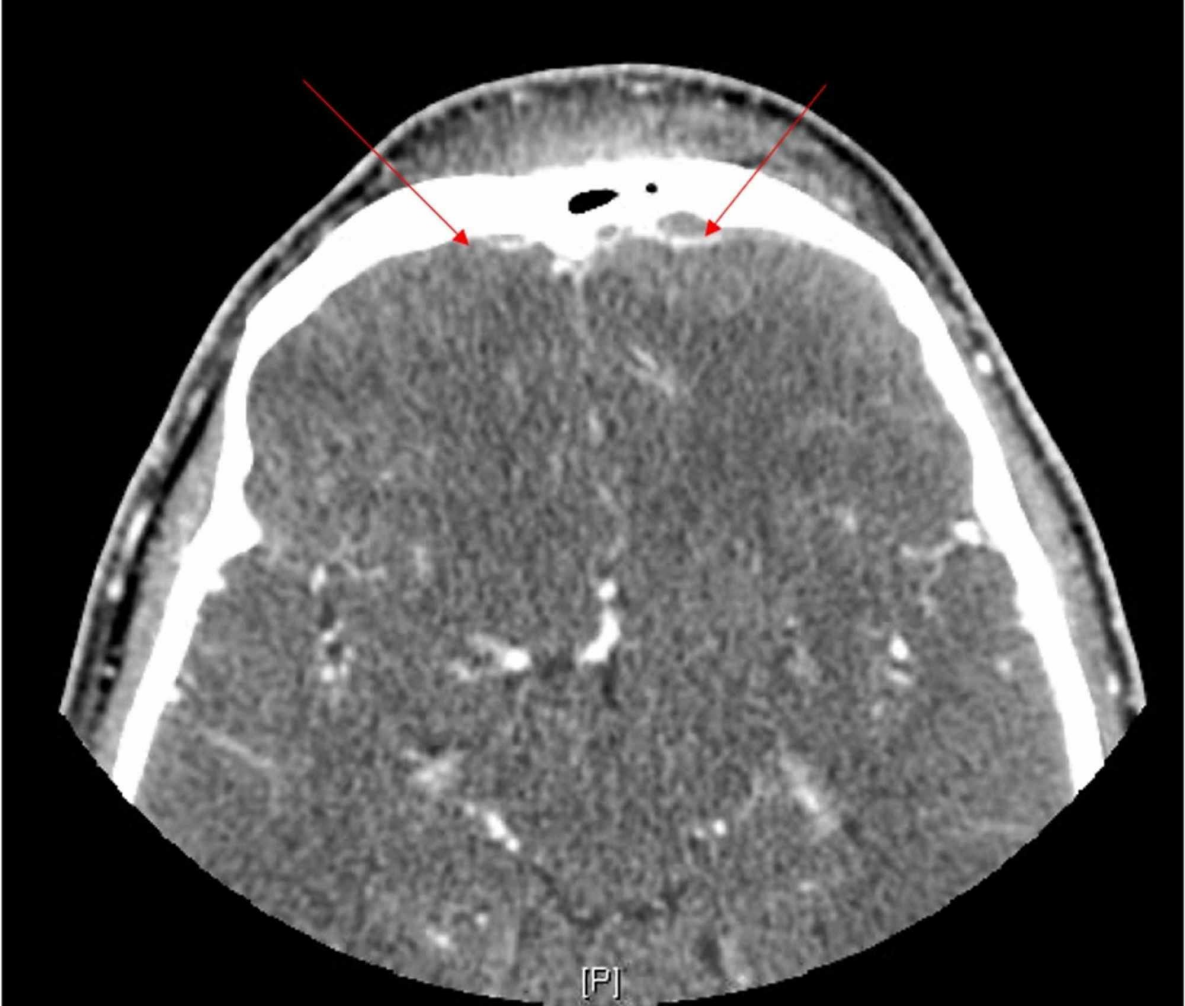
CT head with contrast shows swelling and enhancement of the front scalp. There are peripherally enhancing extra-axial abscesses subjacent to the frontal bones (arrows).
